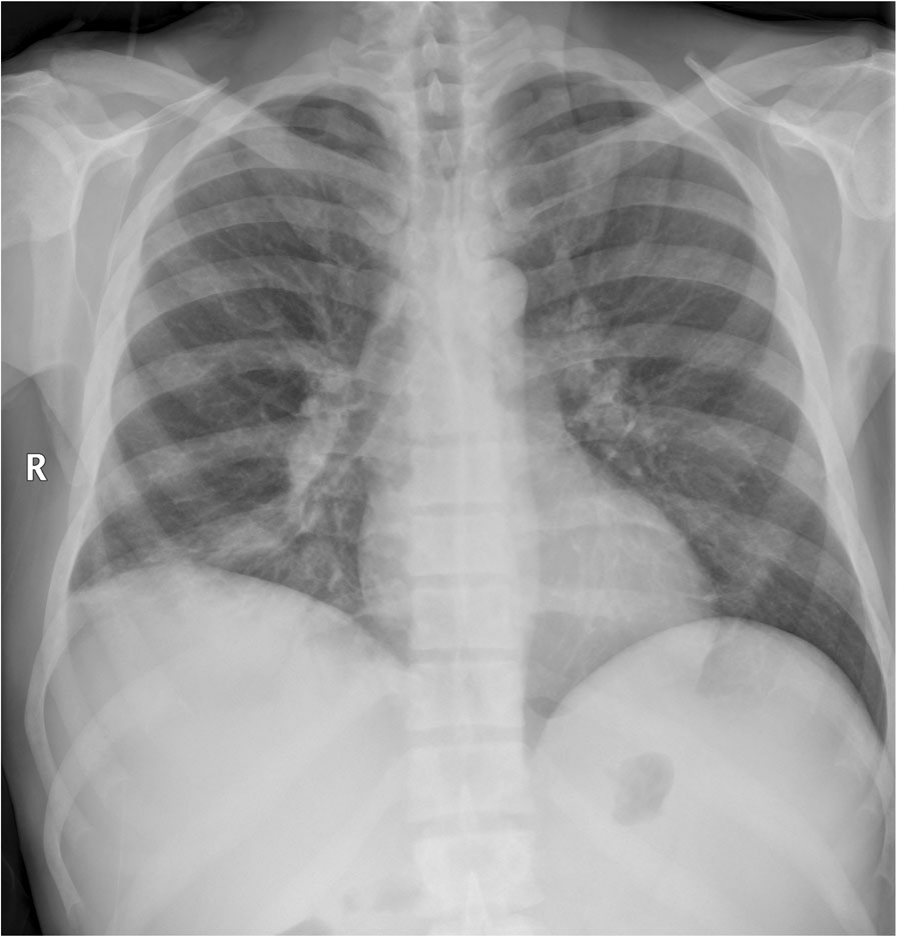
Chest radiograph demonstrating diffuse osteosclerosis in a patient with myelofibrosis. The symmetrical and diffuse appearances of osteosclerosis on plain radiographs are often subtle and difficult to identify
